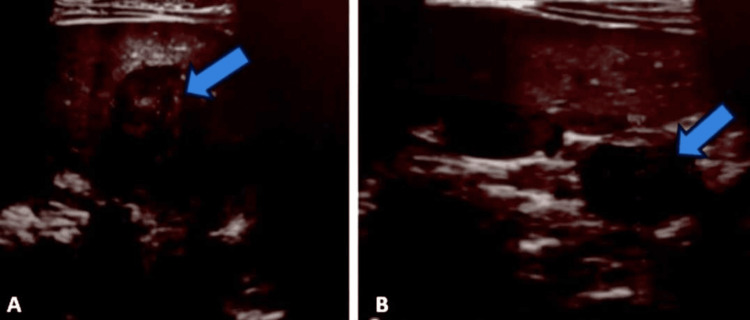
Abdominal ultrasound (A and B) showing splenomegaly with several well-limited, hypoechoic, heterogeneous, rounded formations containing isoechoic areas of variable size, with the largest measuring 32 × 24 mm.
